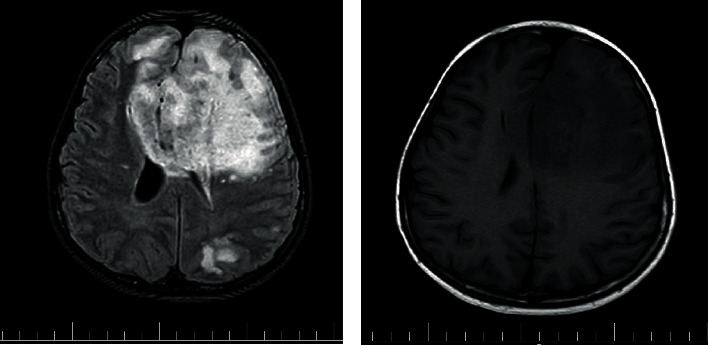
Brain MRI: diffuse abnormal signal foci had seen in both hemispheres, and the largest one is in the left frontal, left temporoparietal, and right occipital regions along with peripheral vasogenic edema.
